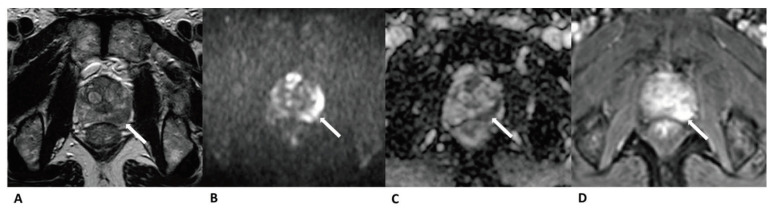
Case of prostate cancer in PZ. Male, 64 years old, prostate cancer with its longest diameter being >1.5 cm. T2WI showed a hypointense nodule in the left PZ (arrow) (A). The lesion was hyperintense on DWI (b = 1500 s/mm^2^) (arrow) and hypointense on the ADC images (B,C). Positive enhancement was seen on the DCE (arrow) (D). This lesion was finally assessed as a PI-RADS category 5, indicating that it had a high cancer risk according to PI-RADS v2, PA PI-RADS v2, and biparametric MRI protocols.
